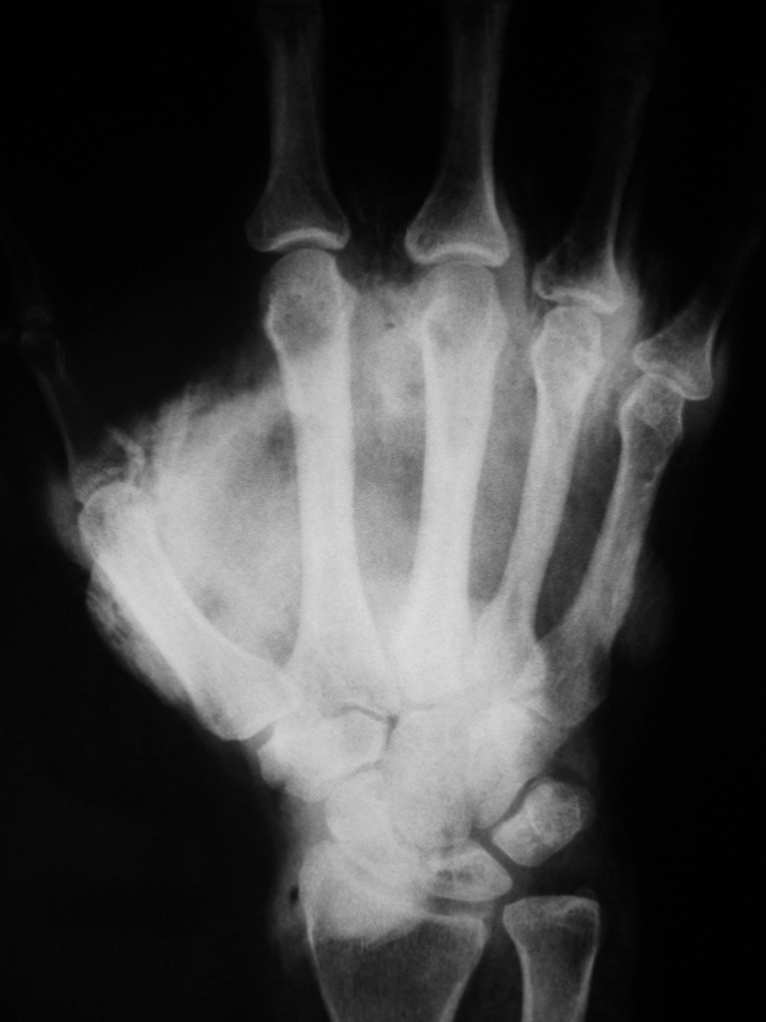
Simple X-ray of the hand that shows a considerable accumulation of extravascular contrast.
